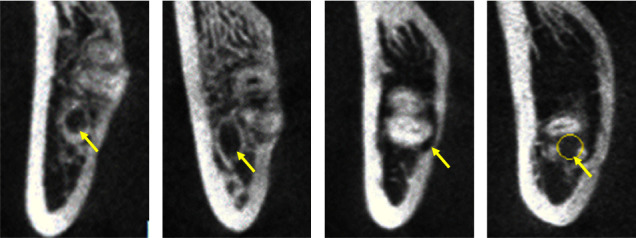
The CBCT images of the mandibular canal at different locations of impacted MTM. The yellow arrow represents the mandibular canal. The yellow arrow represents the mandibular canal. (a) Located below the root; (b) located on the buccal side; (c) on the lingual side; (d) located between roots.
